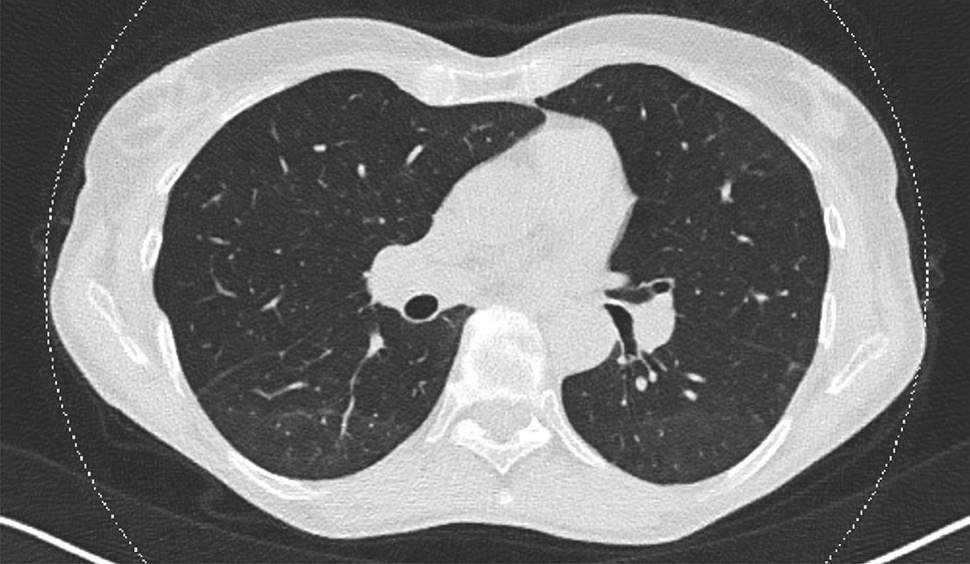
6-month follow-up chest computed tomography scan demonstrating clear regression of pulmonary alterations
